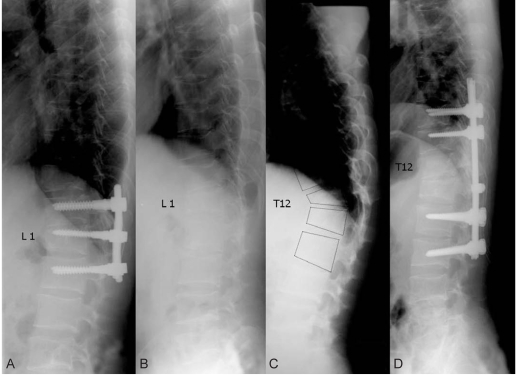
T-L spine lateral radiograph. He had been treated for an unstable L1 burst fracture using posterior instrumentation on the T12-L2 and anterior interbody fusion with autoiliac bone graft 3 years ago (A), and one year ago he had the posterior instrument removed (B). There was a burst fracture with distraction force on T12 (C), and he underwent in situ posterior pedicle instrumentation on the T10-L3 (D).
